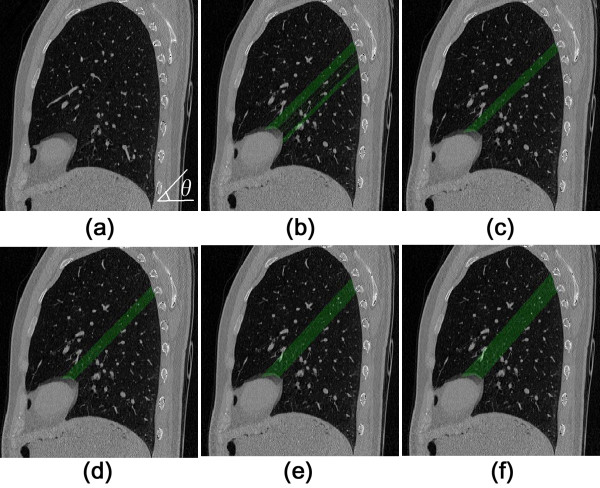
Sagittal adaptive fissure scanning. (a) original sagittal CT image; (b) multiple connected regions at *θ* = 44.22^o^; (c) connected region *CR* with the maximum area at *θ* = 44.22^o^; (d)*CR* with the maximum area at *θ* = 45.62^o^; (e)*CR* with the maximum area at *θ* = 46.95^o^; (f) The final *FR* with the maximum *P* -value.
